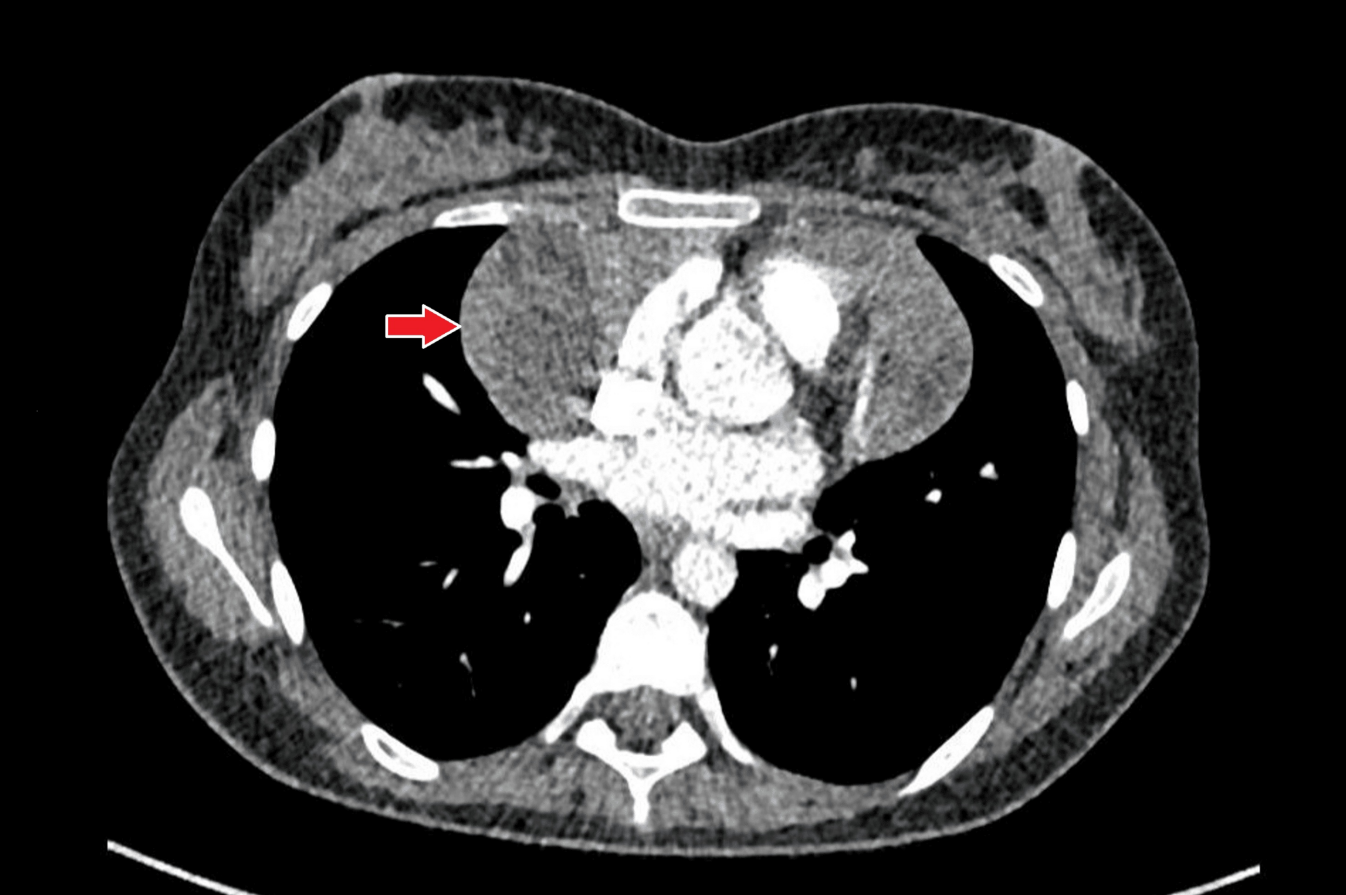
Chest CT angiography demonstrating an anterior mediastinal mass
